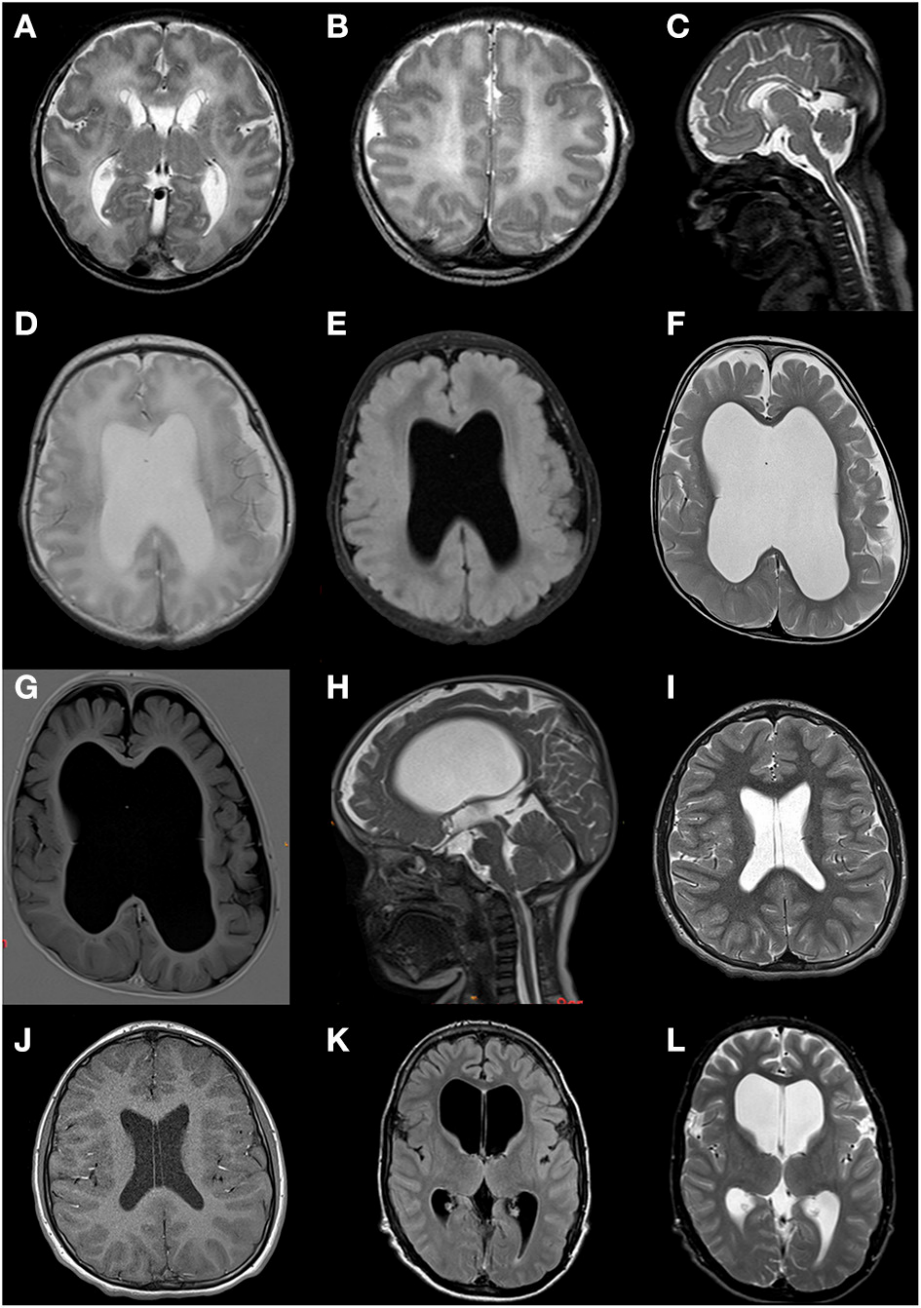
Brain MRI scans of patients 2, 3, 7, and 9. (A–C) Patient 2 at age 2 days; fetal gyral pattern with simplified sulcation, thin corpus callosum with hypoplastic splenium, widened ventricles and cisternae, subependymal pseudocysts, and a cavum septum pellucidum. (D,E) Patient 3 at age 4 days; moderate ventriculomegaly, absence of septum pellucidum, limited volume of the corpus callosum and periventricular white matter, suggestion of cortical malformation of the left temporoparietal region. (F,G) Patient 3 at age 25 months; ventriculomegaly (lateral and third ventricle) and enlarged subarachnoid space due to progressive loss of supratentorial white and gray matter volume; absence of septum pellucidum. (H) Patient 3 at age 28 months; severely enlarged lateral and third ventricle, narrow aqueduct and fourth ventricle, suggesting not only *ex vacuo* dilatation but dysfunction of cerebrospinal fluid. (I,J) Patient 7 at age 12 years; normal MRI but with persistent cavum septum pellucidum and vacuum vergae. (K,L) Patient 9 at age 15 years; marked ventriculomegaly (especially lateral ventricles).
